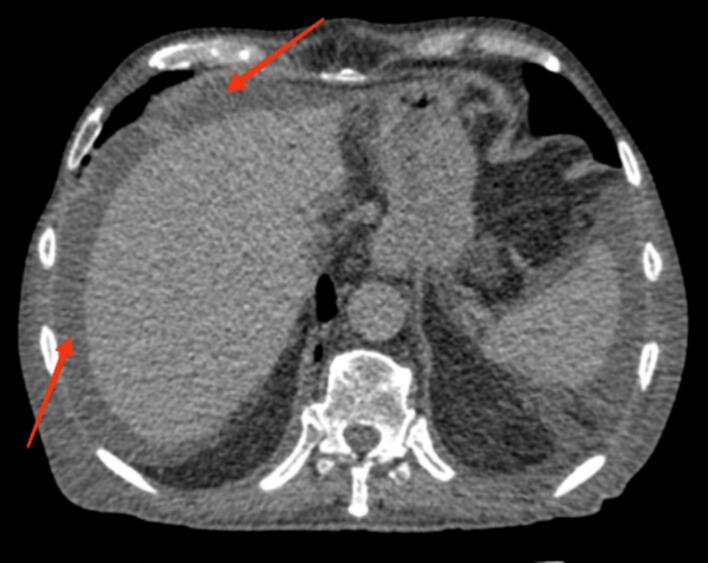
Axial CT scan demonstrating ascitic fluid accumulation in the upper abdomen, with evidence of the fluid surrounding the liver and other abdominal structures.
